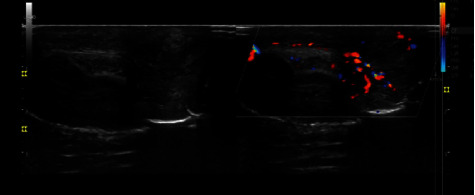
Ultrasound of the penis showing increased color doppler signal on the left side of the penile shaft compared to the right side of the shaft.
